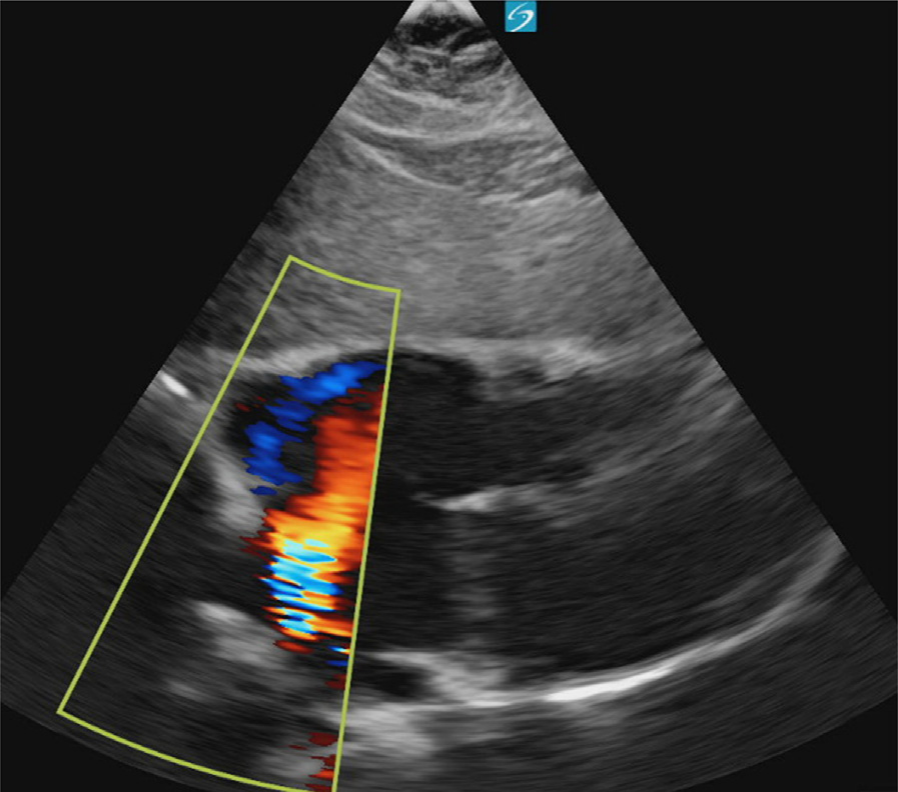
Subxiphoid view with color Doppler revealing right heart dilation and flow across the atrial septal defect. Image taken by the author.
